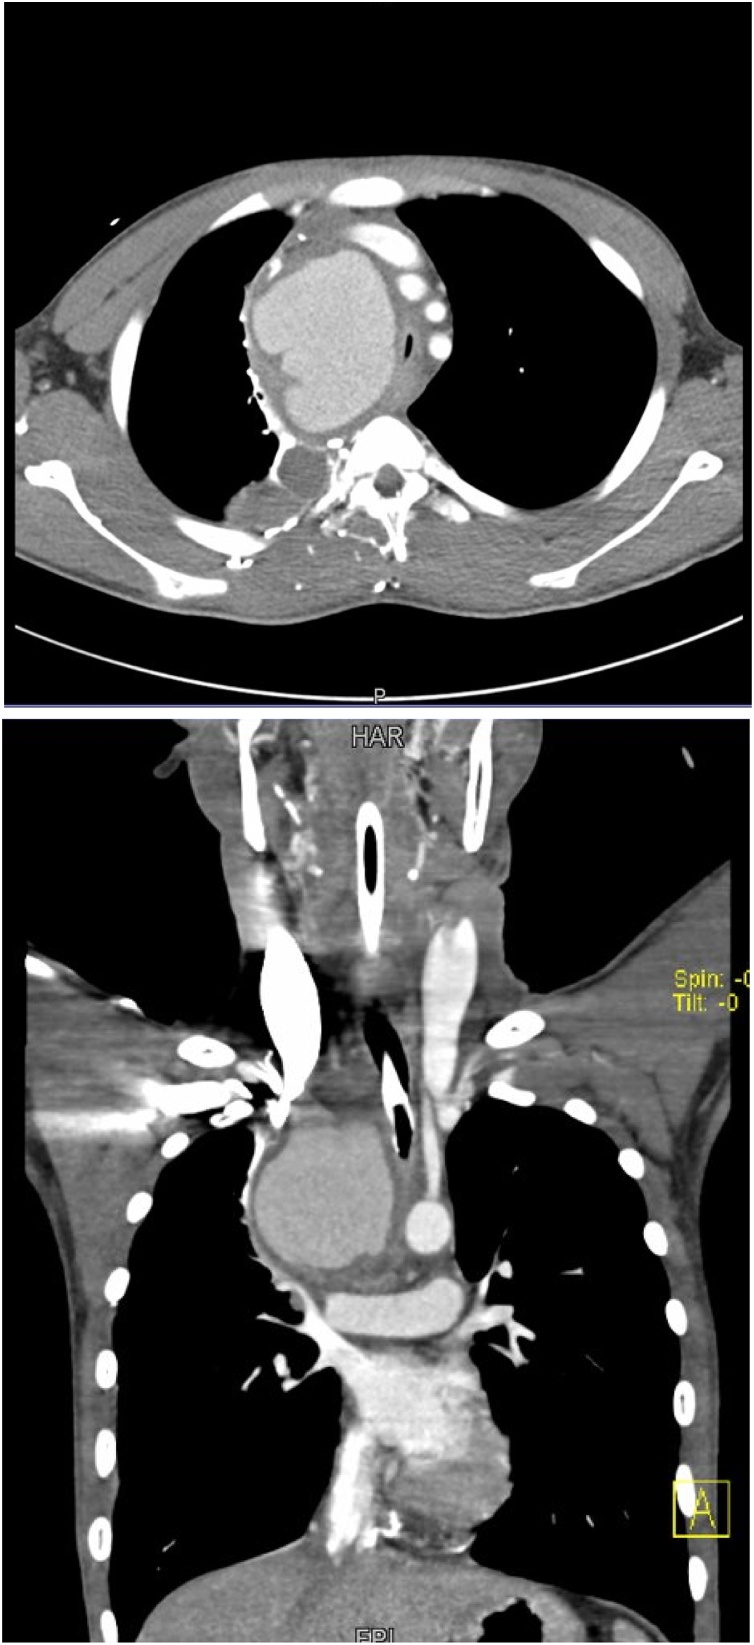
CT chest with IV contrast showing a huge mediastinal vascular mass compressing the airways significantly with contralateral mediastinal shift.
